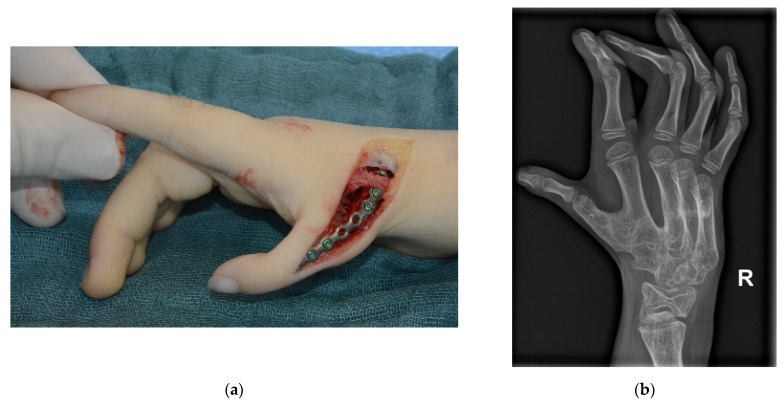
(a) Intraoperative picture of a bone graft interposition after plate fixation (b) X-ray of a long term follow up after bony consolidation and plate removal.
